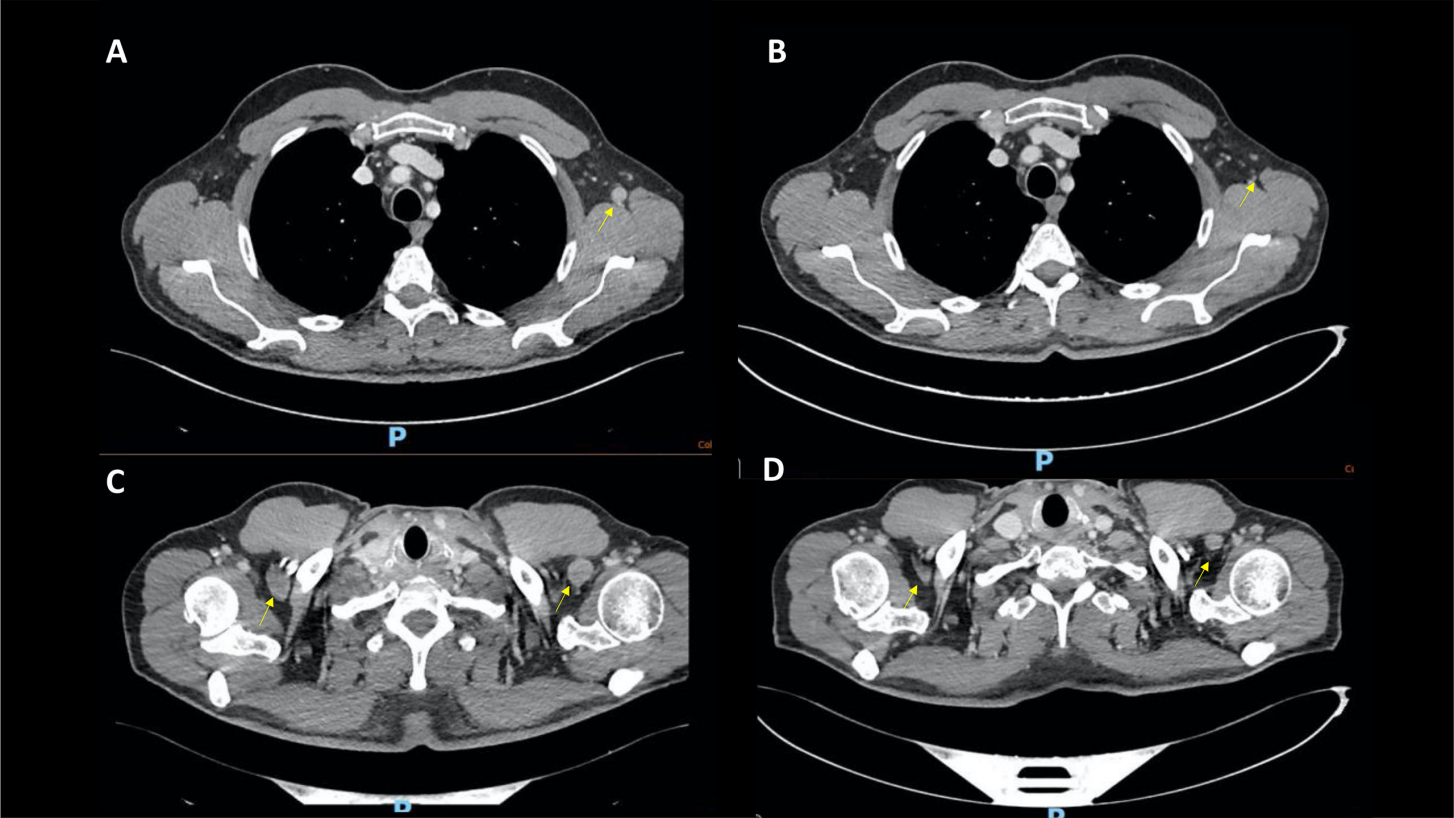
Case 2, CT scans showing significant shrinking in the axillary lymph nodes from December 2021 (A, C) to April 2022 (B, D).
